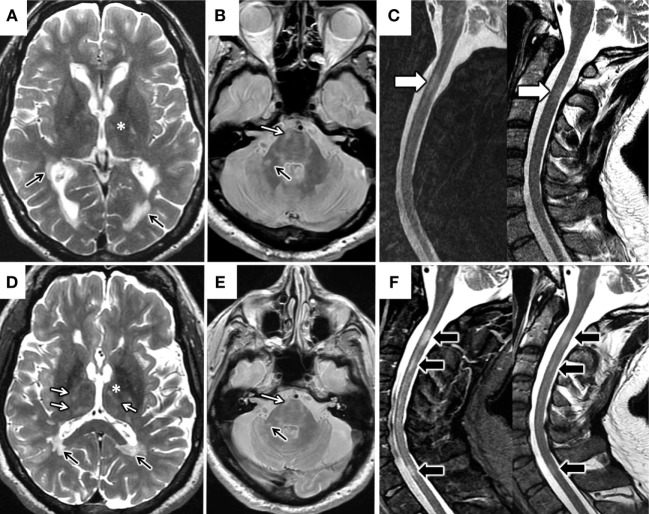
Changes in brain and spinal cord MRI. MRI 2010 (A–C). Axial T2 FSE MRI image of the brain (A) demonstrates multiple periventricular lesions in the lateral ventricles (black arrows) and scarce thalamic lesions (*). Axial proton-density-weighted MRI image (B) shows predominant infratentorial lesions (white arrow in pons and black arrow in right middle cerebellar peduncle). Sagittal STIR and T2 FSE MRI images of the spinal cord (C) highlight the presence of a short segment spinal cord lesion (white arrow in C2). MRI 2016 (D–F). Axial T2 FSE MRI image (D) shows increased in the number of thalamic lesions (* and white arrows) and stability of the periventricular lesions (black arrows). Axial proton-density-weighted MRI image (E) shows stability of the infratentorial lesions respect to 2010 (arrows). Sagittal STIR and T2 FSE MRI images of cervical spinal cord (F) demonstrate new short segment lesions affecting C3 and C7 levels respect (black arrows).
